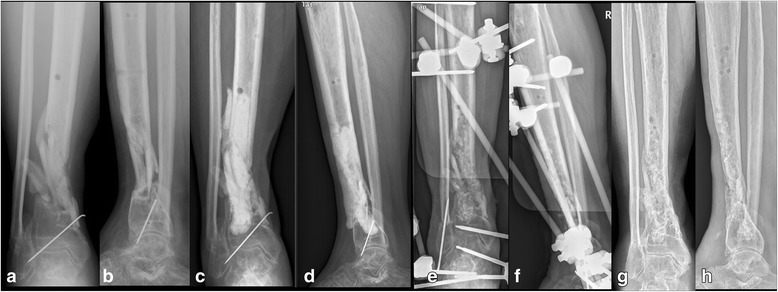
A 42-year-old male suffered osteomyelitis of the right distal tibia. X-ray films on admission showed nonunion of the tibia (a-b). A thorough debridement was performed. Segmental bone defect occurred after debridement. The bone defect was filled with a cement spacer and the lower limb was stabilized with a plaster cast (c-d). Sixty-two days later, the cement spacer was removed with preservation of the induced membrane, cancellous autografts were placed within the induced membrane, and the tibia was stabilized with a standard external fixator (e-f). The bone was healed at 8 months after the bone grafting; radiographs at 1.5 years follow-up showed bone union (g-h)
